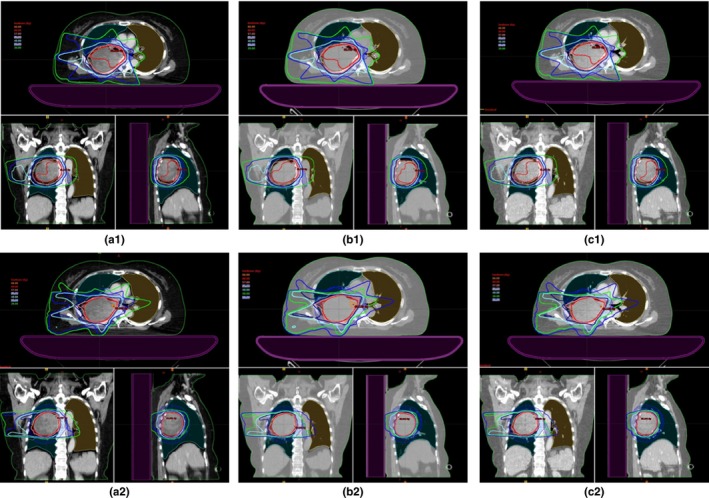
Isodose distributions in a patient as shown by three types of CT imaging of AIP images plan (a1 & a2), FB image plan (b1 & b2), and MidV image plan (c1 & c2) in 3DCRT (top row) and IMRT (bottom row). Orange = 66 Gy, red = 60 Gy, pink = 57 Gy, dark blue = 50 Gy, cyan = 40 Gy, blue = 30 Gy, green = 20 Gy.
